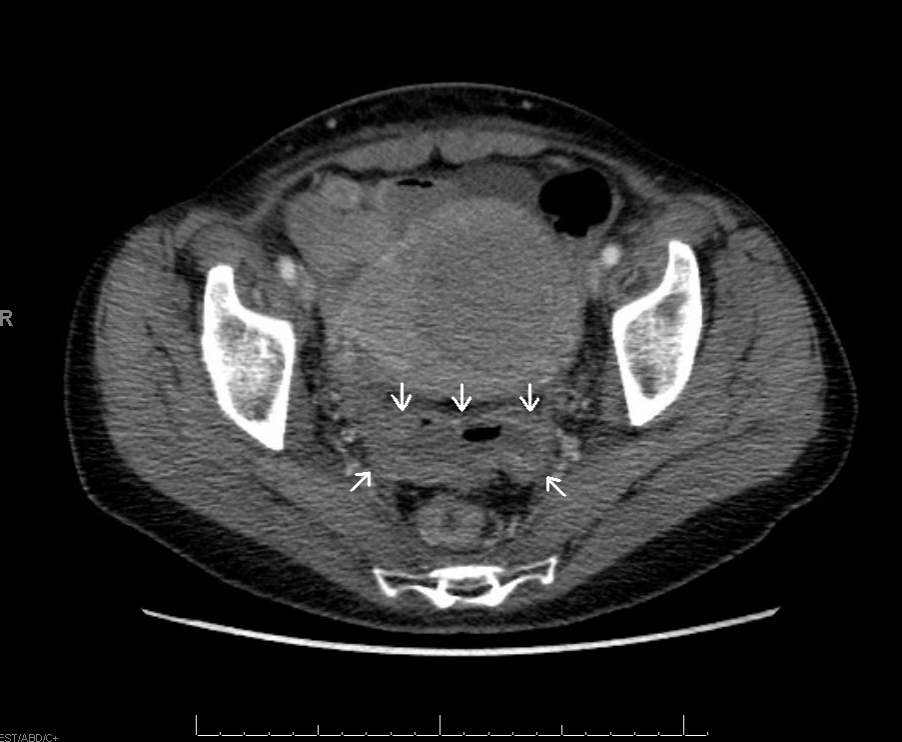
CT-scan transverse image showing thickening of the sigmoid wall
